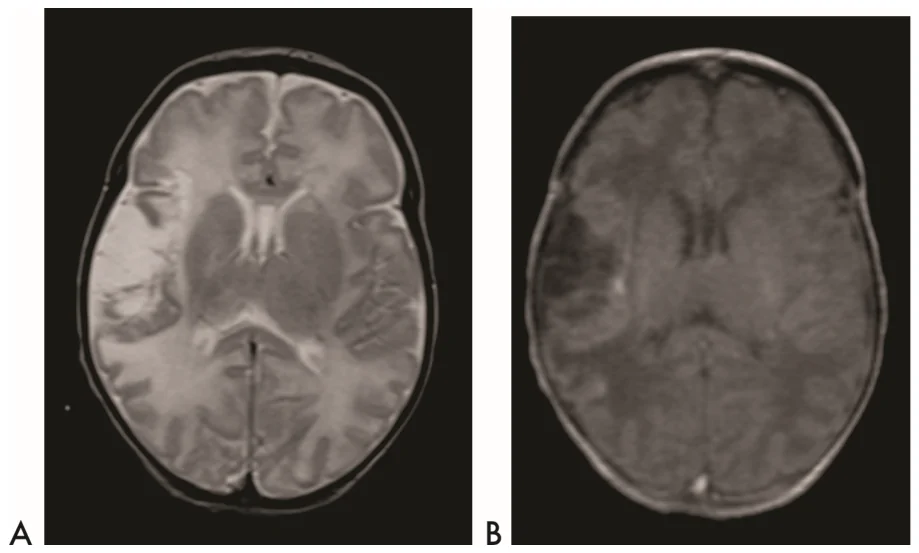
Case: MRI day 17. (A). T2 imaging demonstrating evolution of the right middle cerebral artery infarct with encephalomalacia. (B). T1 imaging demonstrating development of right-sided laminar necrosis vs. microhemorrhage and resolution of previously seen left basal ganglia hemorrhage.
